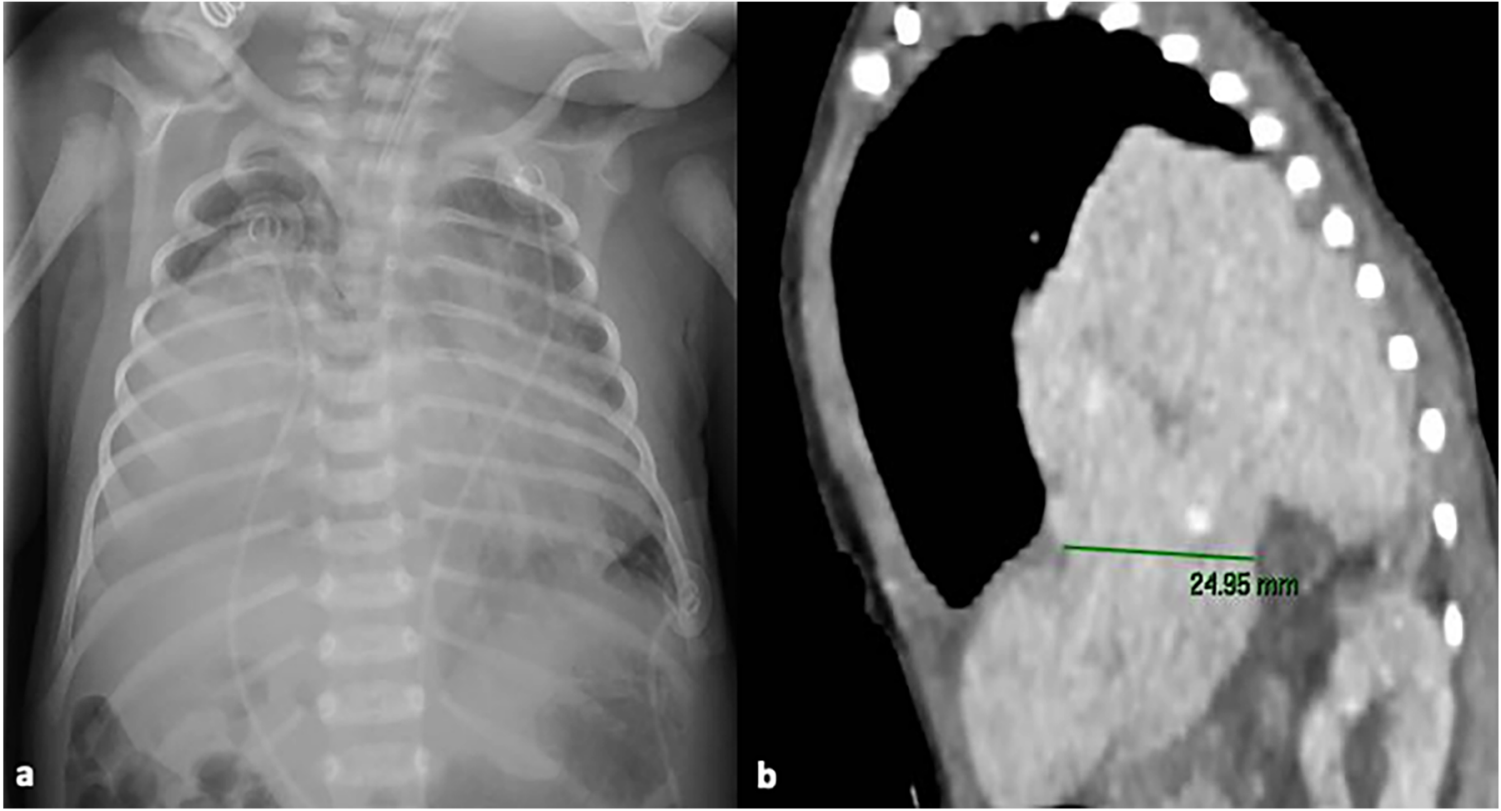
Chest x-ray at NICU admission showing areas of consolidation in both lung fields (a); chest computed tomography scan, showing the presence of a posterior CDHR, through a diaphragmatic defect measuring approximately 25 mm: the liver was herniated into the intrathoracic space, reaching up to the middle-upper third of the right lung field (b) written informed consent was obtained from the patient.
